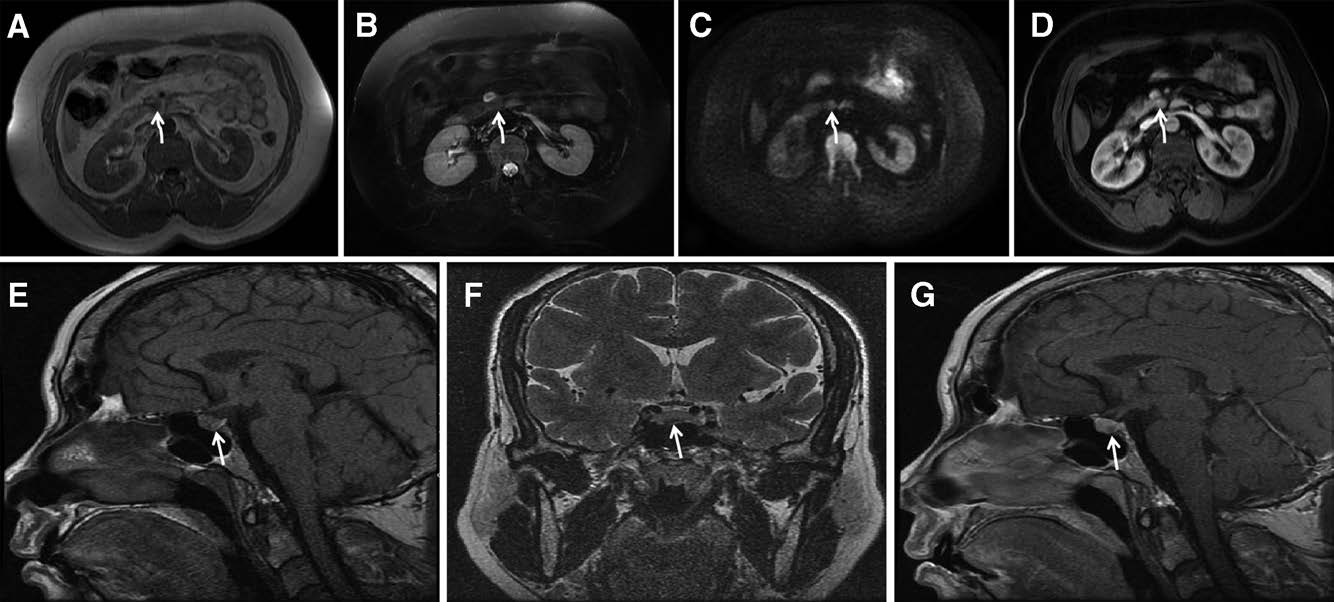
Upper row (A–D): MR study of the uncinate process of the pancreas. Lower row (E–G): MR study of the pituitary. (A) A small nodule with slightly low signal on T1WI. (B) Identical signal on T2WI. (C) Slightly high signal intensity on DWI. (D) The lesion was with minimal enhancement. (E–G) Identical signal on T1WI and slightly low signal on T2 in the right lower pituitary, the enhanced scan revealed a hypointense lesion. MR = magnetic resonance, T1WI = T1-weighted imaging, T2WI = T2-weighted imaging.
